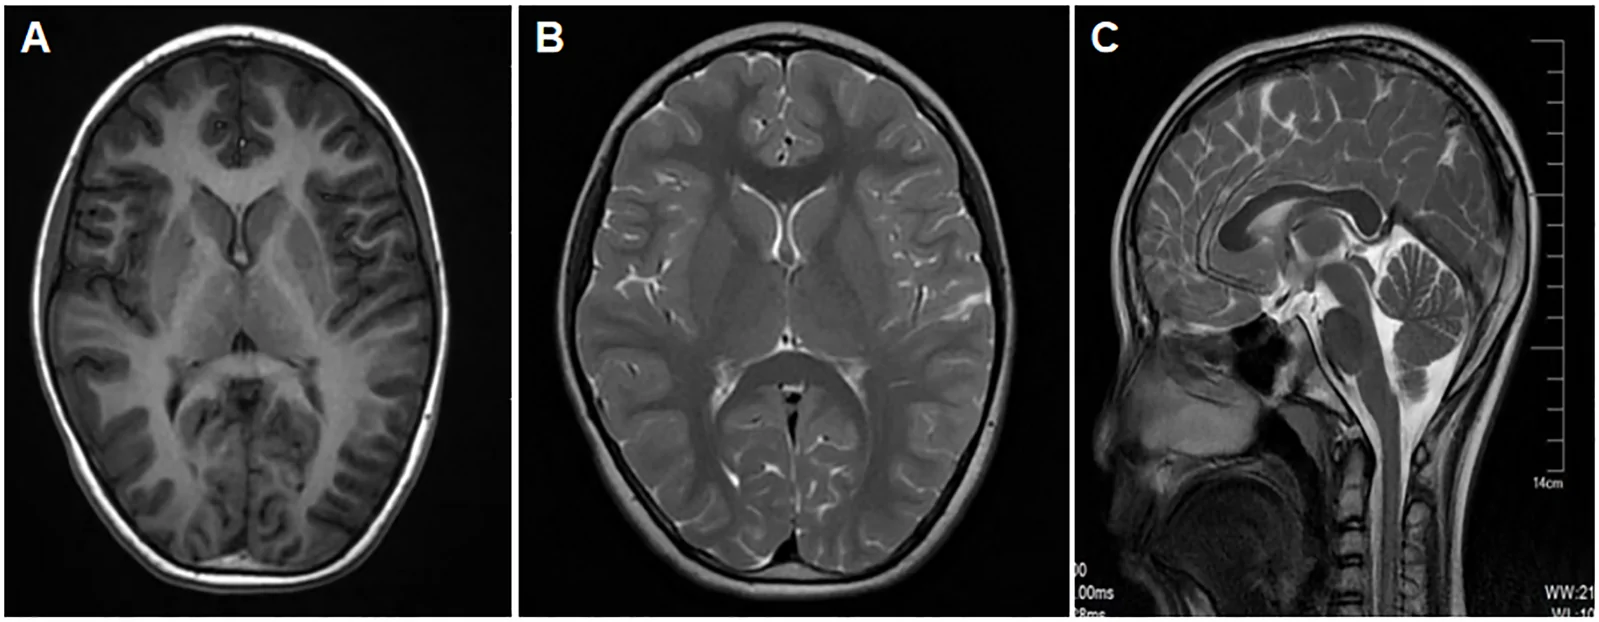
MRI brain scan revealing no significant abnormal signals, (A) T2 image of the axial section, (B) T2 flair image of the axial section, and (C) T1 image of the sagittal section.
